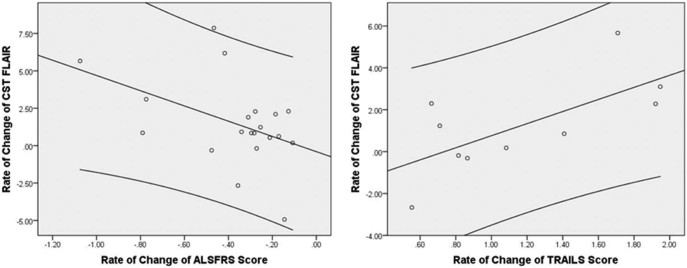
Regression analysis of rate of change of CST FLAIR intensity over time vs rate of decline of ALSFRS (R^2^ = 0.198, *P* = 0.05) and vs rate of change in trail making test, B − A score (R^2^ = 0.458, *P* = 0.03). x axis: rate of change of clinical score per month of disease; y axis: rate of change of MRI intensity per month of disease × 10^−3^. Left panel: A single outlier with a very high rate of ALSFRS-R decline >2 points per month was excluded. Right panel: Only 10 patients were able to complete the TMT on their second visit, hence the smaller dataset. ALSFRS-R, revised Amyotrophic Lateral Sclerosis Functional Rating Scale; CST, corticospinal tract; FLAIR, fluid-attenuated inversion recovery; MRI, magnetic resonance imaging; TMT, Trail Making Test.
